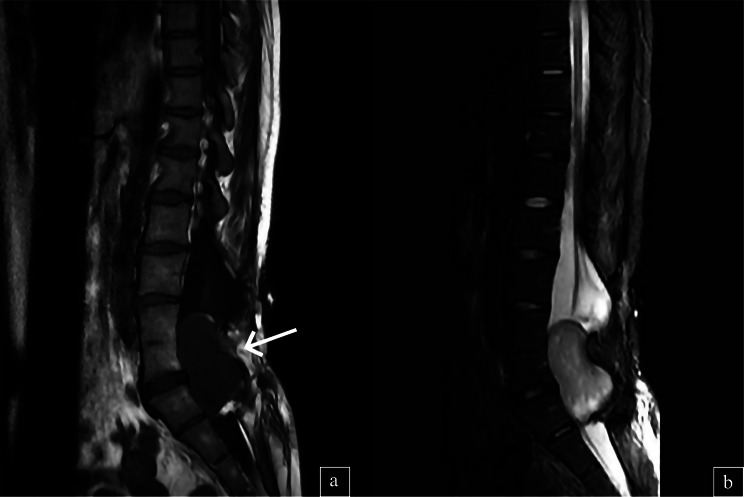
(a) T1-weighted lumbar MRI image showed a well-defined hypointense mass (arrow) within the spinal canal at the L4-S1 level. (b) In the sagittal T2-weighted MRI image, the lesion measuring 6 × 5 × 3 cm appeared hyperintense
